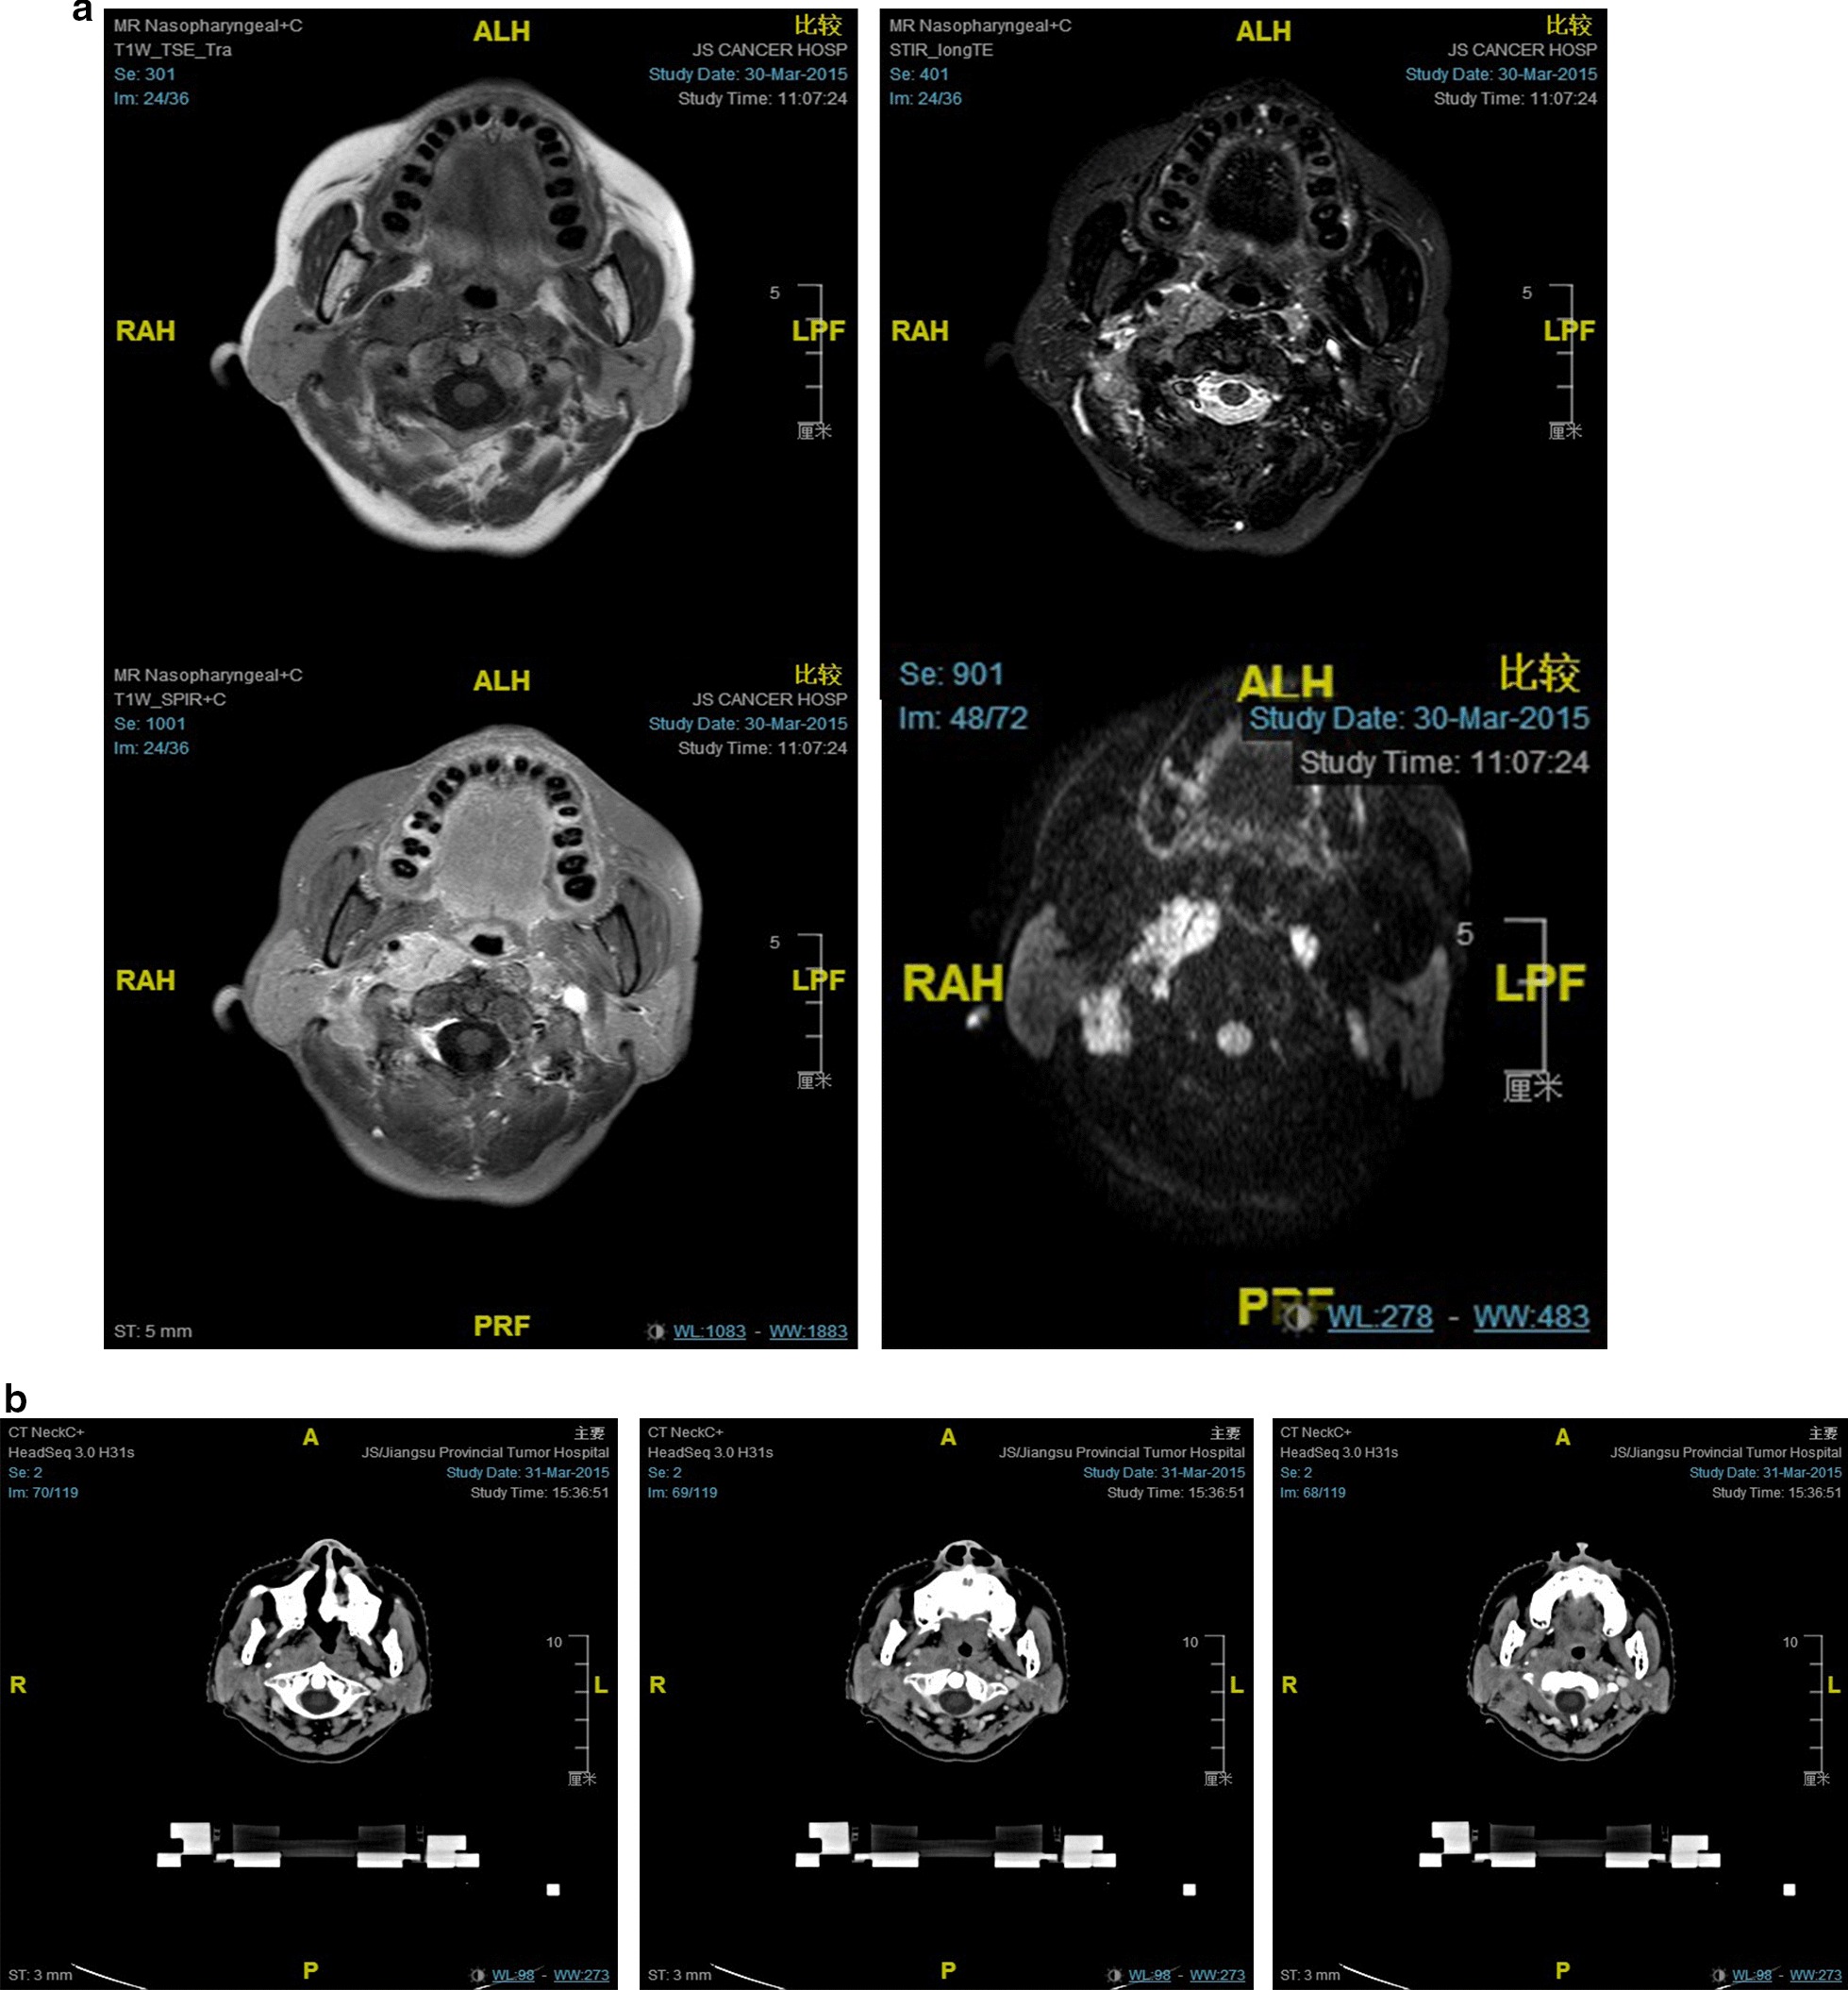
A case with an involved lymph node superior to the upper border of level IIb. a MRI (T1-weighted, T2-weighted, T1 C+ and DWI). b Three successive slides from a planning CT
